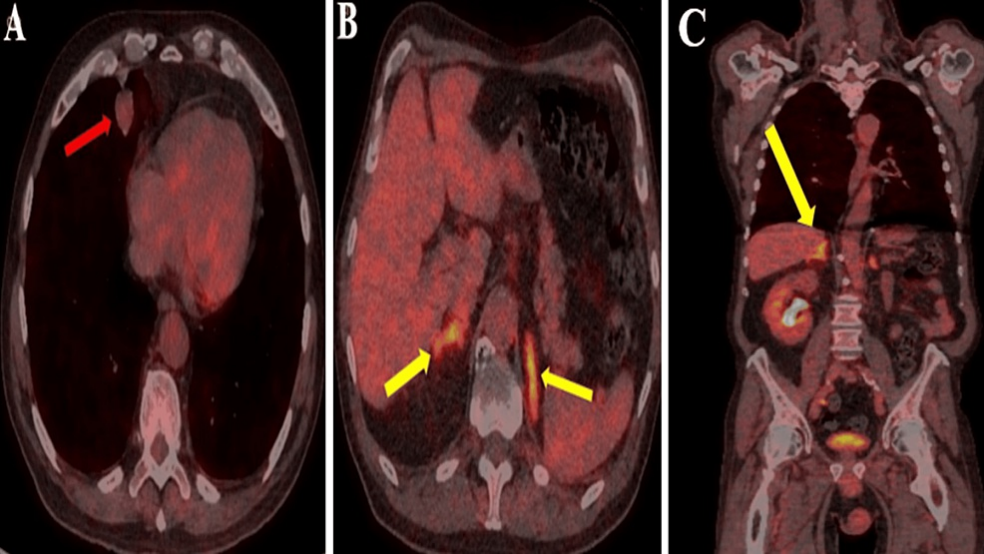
Axial fluorine18-fluorodeoxyglucose positron emission tomogram computed tomogram (FDG PET CT). (A) Right middle lobe pulmonary nodule with low-grade uptake of activity (Herder faint) (red arrow). (B, C) Axial and coronal FDG PET CT images demonstrating high-grade uptake within bilateral prominent adrenal glands with no discrete nodules (yellow arrows).
